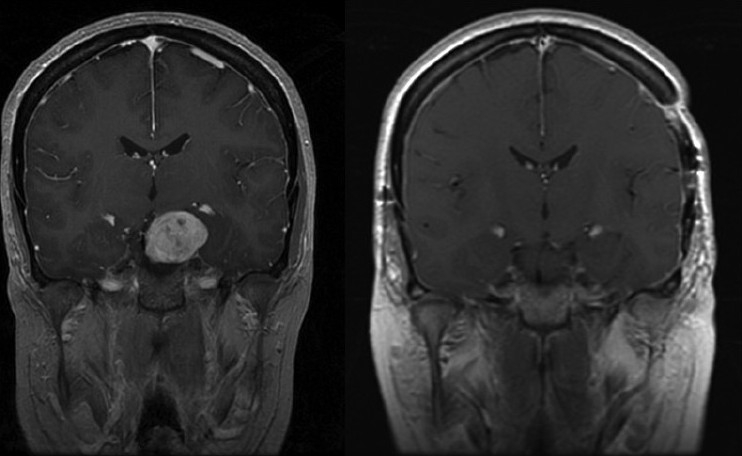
A coronal gadolinium-enhanced contrasted T1-weighted MRI scan showing a large parasellar/ suprasellar mass with significant brainstem compression (a). A postoperative coronal gadolinium-enhanced T1-weighted MRI contrast scan 3 months later showing a complete resection of the mass with brainstem compression reversed (b). A combined subtemporal and anterior Sylvian fissure-splitting approach was used to reach the tumor
